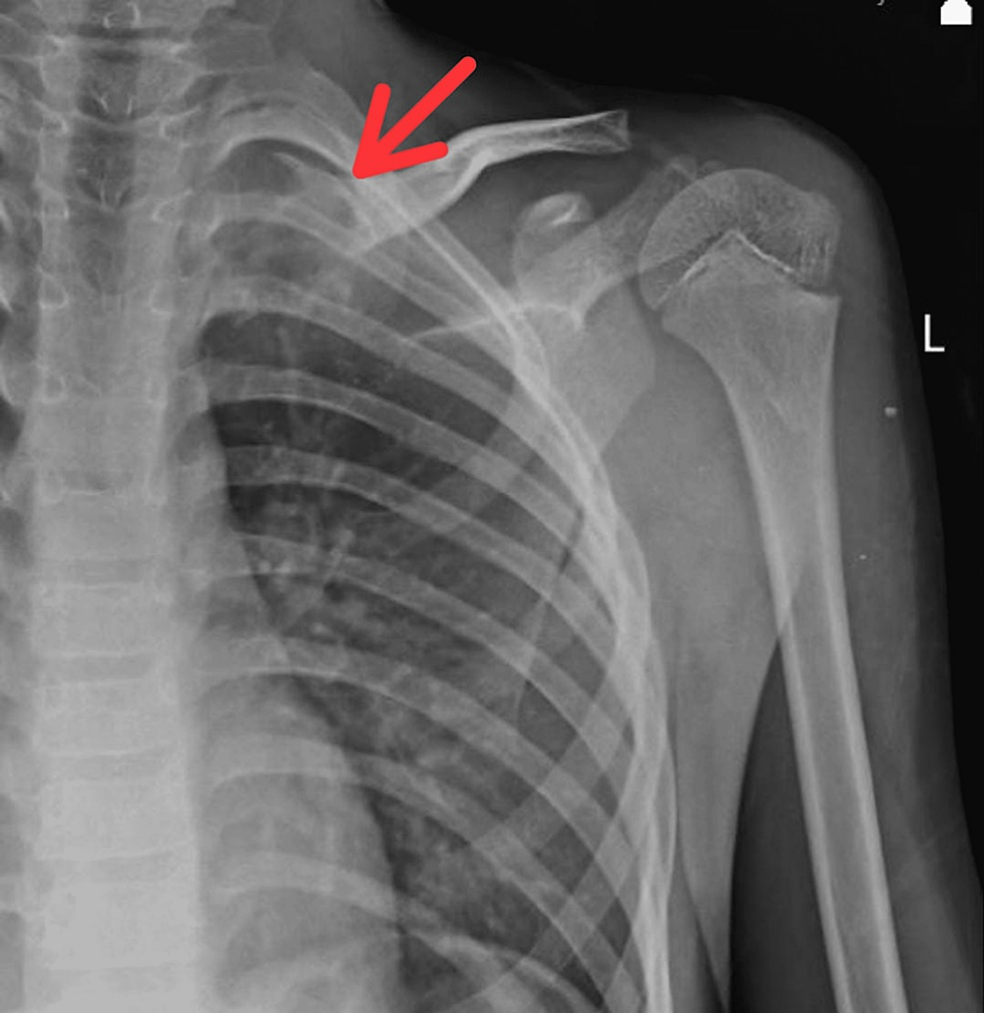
Pre-operative X-ray of the left shoulder with clavicle showing lesion over medial end of clavicle
